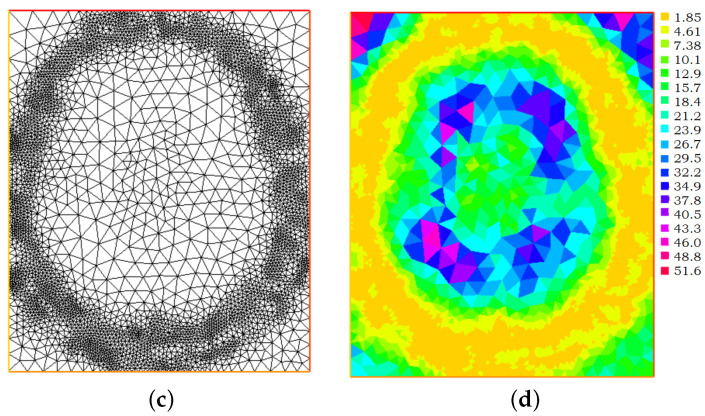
Treatment of the T2-weighted MR scans. (a) G for image id N22, γ=1/8; (b) isolines of the linear finite-elements approximation; (c) final resulting triangulation; (d) area of the triangles in the final triangulation.
